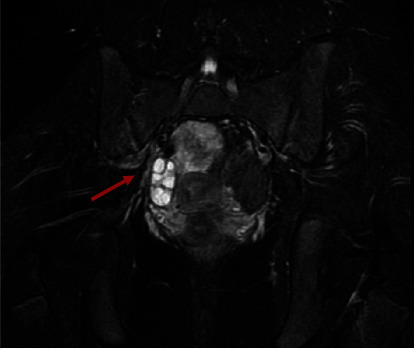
The MRI showed liquid in the Douglas cavity and slight asymmetry of the contrast impregnation in the early phase of the right ovary (arrow) which appeared more visible in the late phase.
